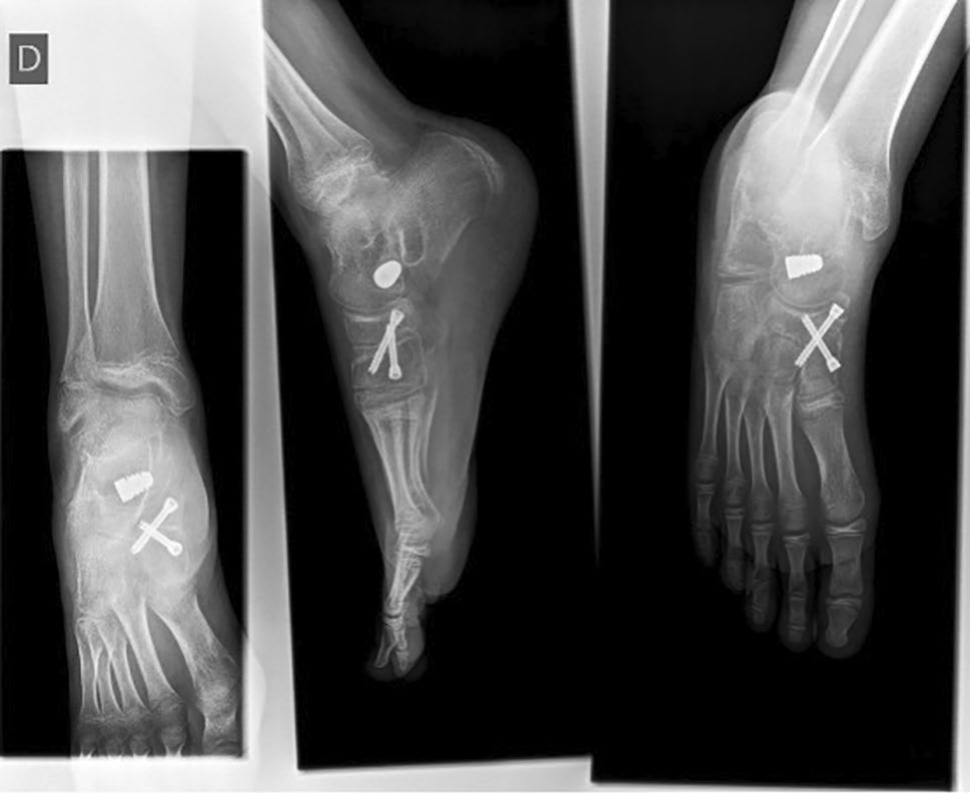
Three months post-surgical x-ray of right foot (antero-posterior, latero-lateral, oblique views)
